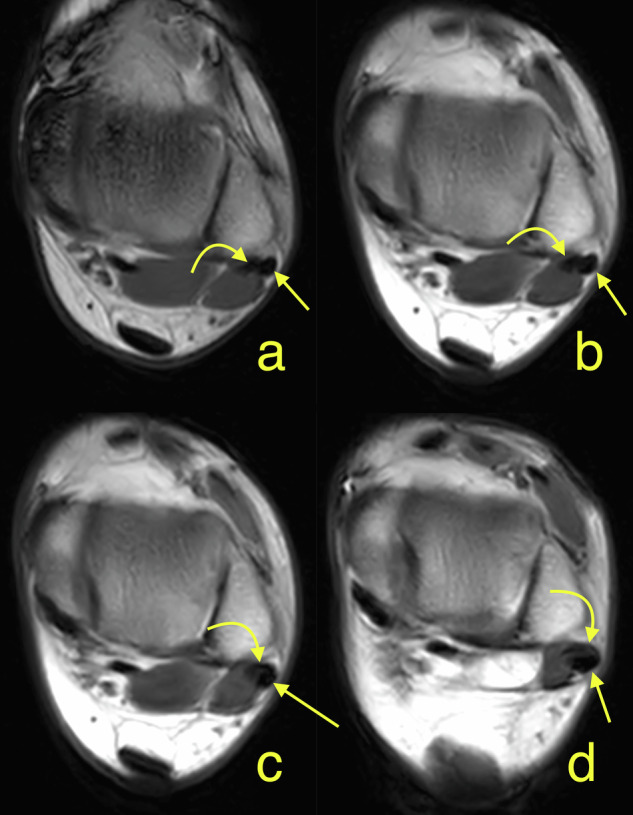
Dynamic 3-T MRI of the ankle at the level of the lateral malleolus, transverse sections. a–d Show sequential frames used to assess peroneal tendon stability, from plantarflexion (a) to dorsiflexion (d). The PB is indicated by a curved arrow, and the PL by a straight arrow
